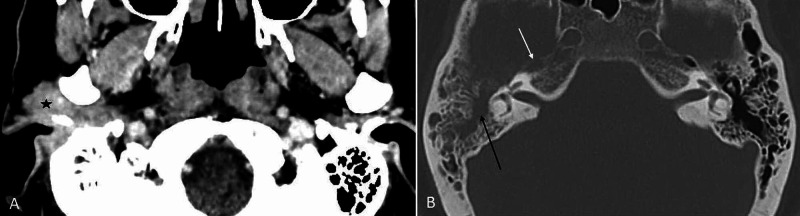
CT of the brain. (A) Contrast-enhanced CT demonstrating an enhancing lesion at the right external auditory canal, pre-auricular region (black star). The ipsilateral mastoid air cells are not aerated filled with soft tissue density, while preserving the bony septation. (B) CT in the bone setting showing the mass occupying the middle ear cavity with the destruction of the incudomalleal complex (black arrow). The anterior cortical margin of the right petrous apex is destructed (white arrow). CT, computed tomography
